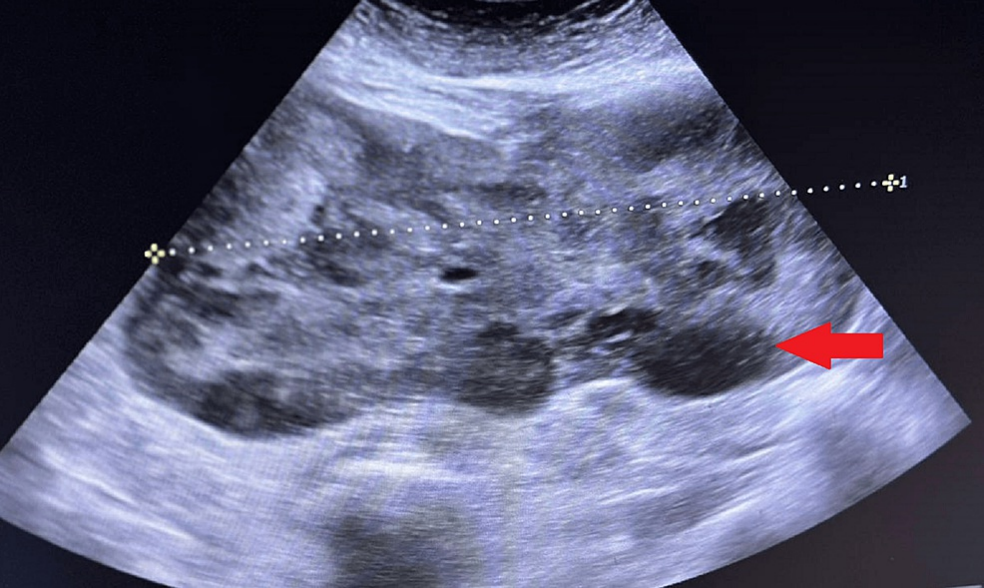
Ultrasonography demonstrating anechoic cystic mass
